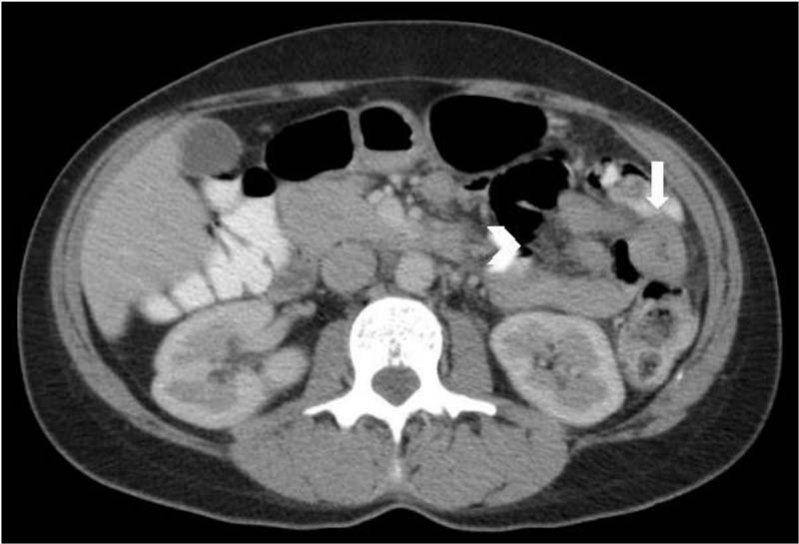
Axial CT abdomen and pelvis with portal venous phase and oral contrast, demonstrating a short segment of thickened jejunum (arrow) in the left upper quadrant with associated mesenteric enhancement (arrow head).
